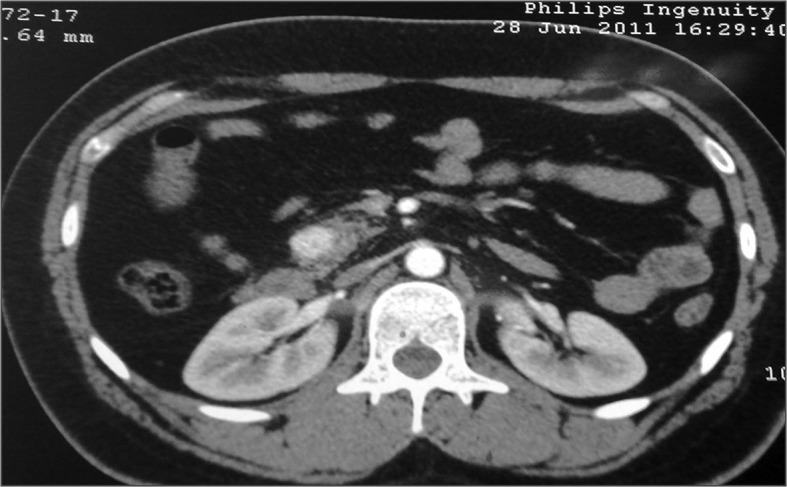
Contrast enhanced CT (transverse section) revealed a solitary insulinoma in the head of pancreas.
